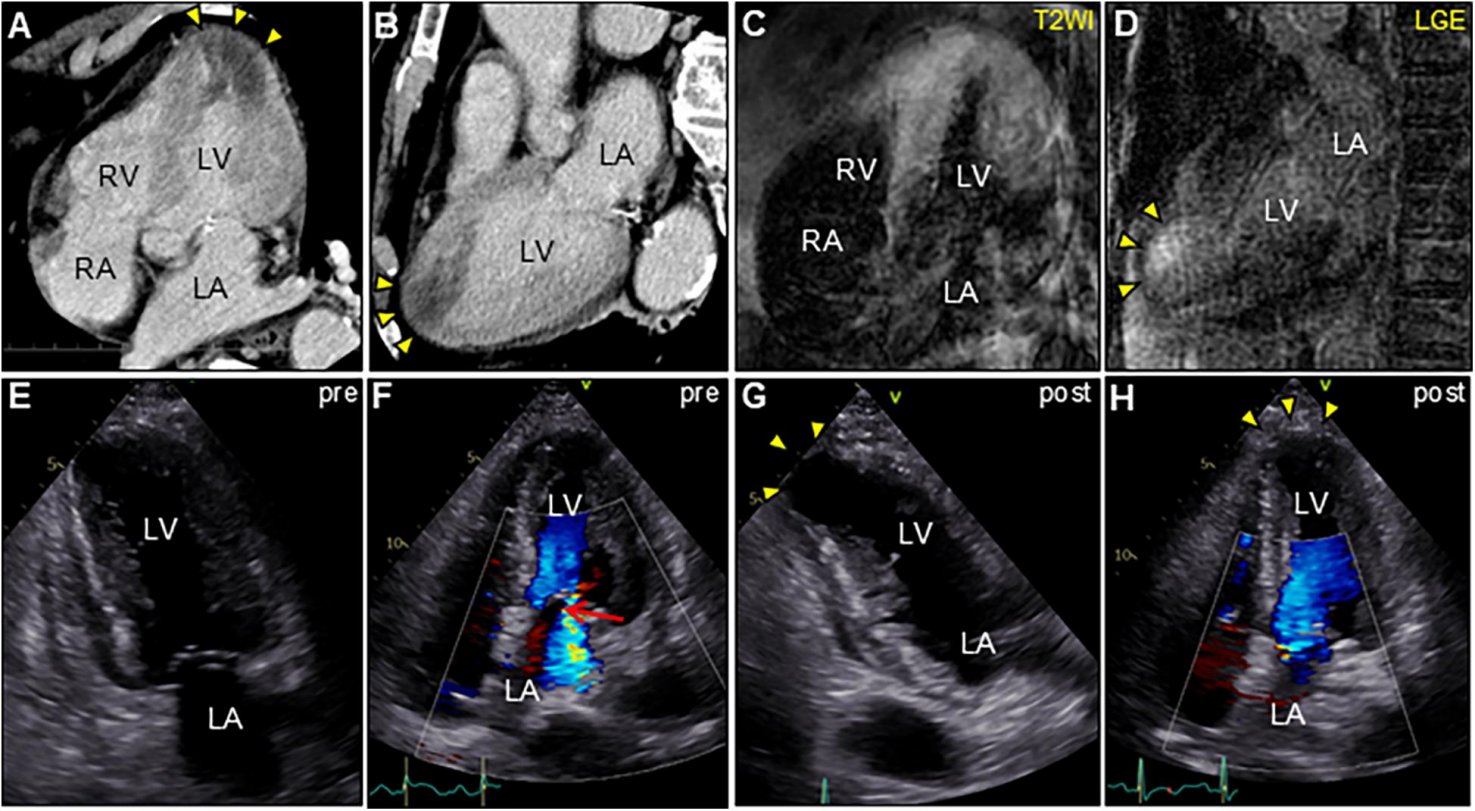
Enhanced computed tomography (CT) and cardiac magnetic resonance imaging (CMR) (A,C, apical four-chamber view; B,D, long-axis view). CT showing low-attenuation areas of the left ventricular apex (arrowheads). T2-weighted image (T2WI) showing diffuse myocardial edema. Late gadolinium enhancement (LGE) images showing the same region as that of CT (arrowheads). Serial transthoracic echocardiography (TTE). (E,G, apical two-chamber view; and F,H, apical four-chamber view). (E,F) TTE on day 6 after admission showing severe global left ventricular systolic dysfunction with increased left ventricular wall thickness (LVWT) accompanied by severe mitral regurgitation (arrow) in color Doppler mode [LVWT, 14 mm; and ejection fraction (EF), 40%]. (G,H) Follow-up TTE 2 weeks after corticosteroid treatment showing considerable improvement in left ventricular function and mitral regurgitation (LVWT, 12 mm; and EF, 57%). Note the left ventricular apical aneurysmal formation (arrowheads). LA, left atrium; LV, left ventricle; RA, right atrium; RV, right ventricle.
